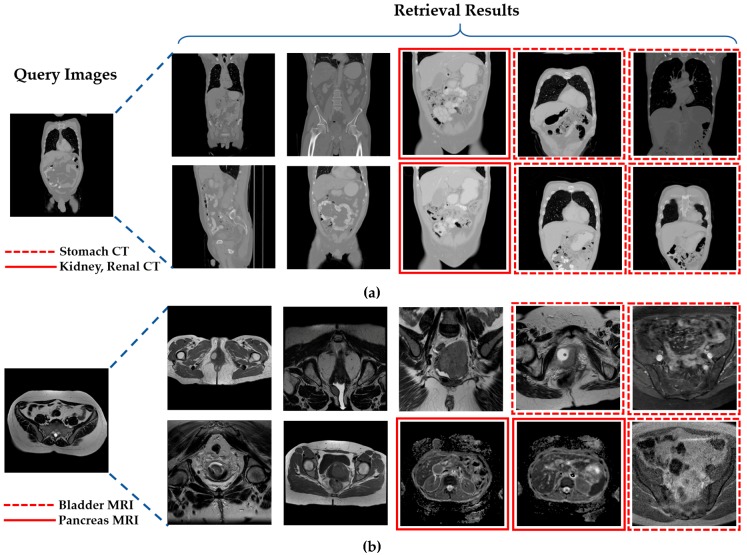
Class-prediction-based false retrieval performance of our proposed system. (a) Bladder CT scan as a query image; (b) cervix MRI scan as the query image.
